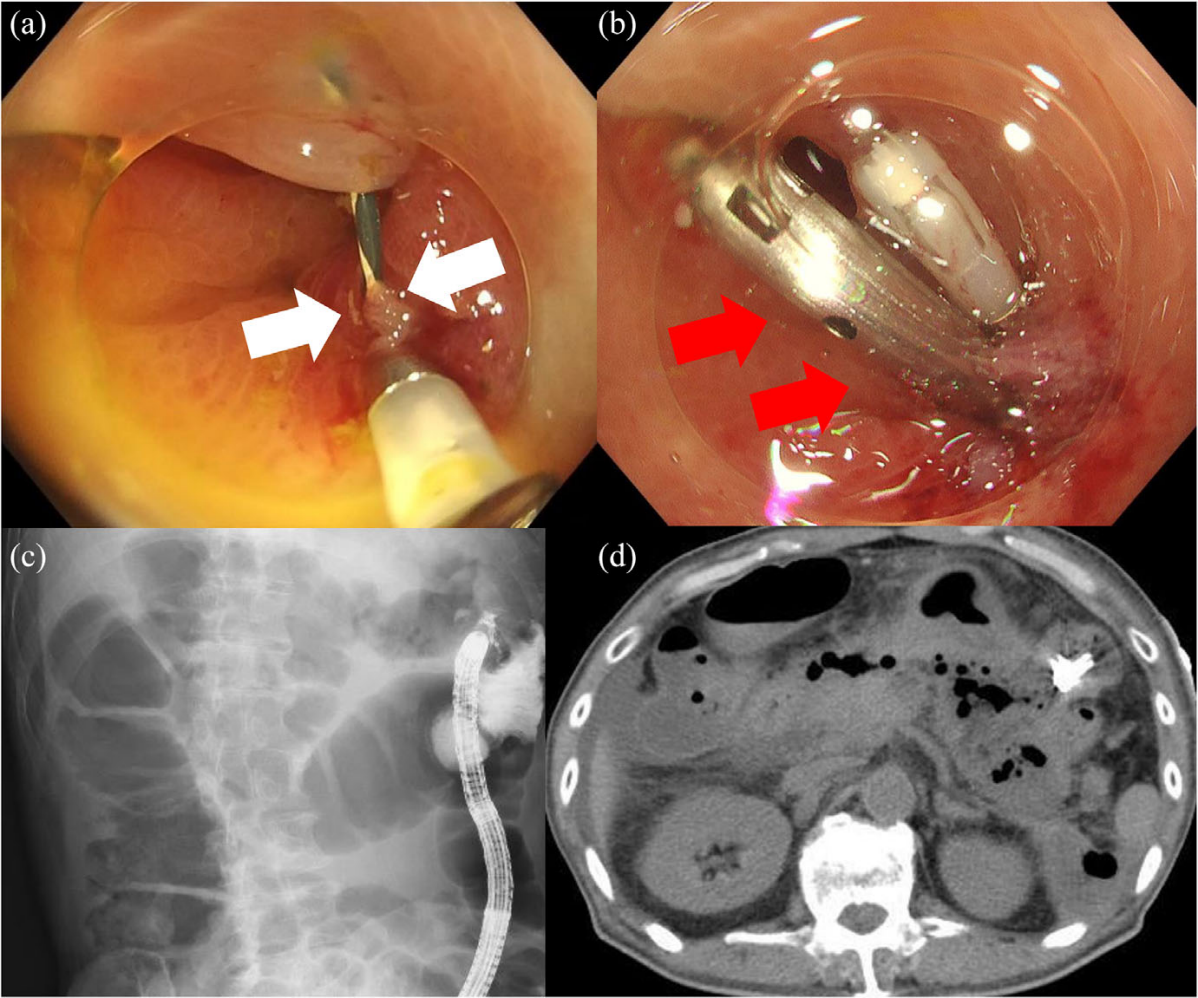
(a) The defect was approximated using an endoclip under full vision, with the guidewire covered with surrounding mucosa. A defect measuring 2 mm in diameter is visible (white arrows). (b) Closure was completed successfully. A MANTIS clip (Boston Scientific Japan; red arrows) was placed to increase the strength of the closure. (c) Contrast imaging confirmed no leakage of contrast media outside the intestinal tract. (d) CT performed the next day showed no evidence of free air or peritonitis. CT, computed tomography.
